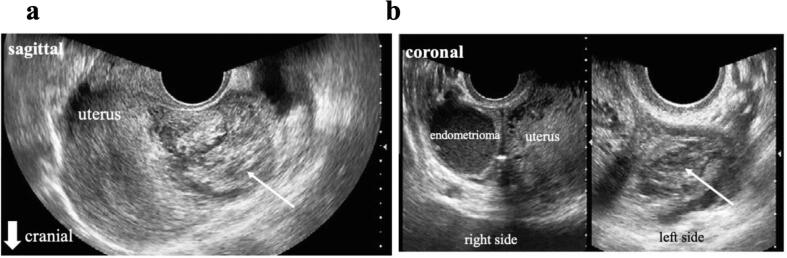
(a) Transvaginal ultrasound indicates an empty uterine cavity with no evidence of a gestational sac, along with the heterogeneous mass (arrow) on the posterior wall of the uterus. (b) Transvaginal ultrasound shows an ovarian endometrioma and the heterogeneous mass (arrow).
